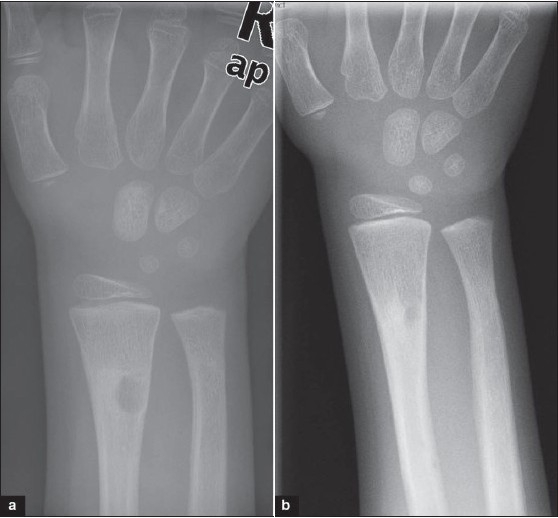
Anteroposterior X-ray (a) of forearm with wrist at 3 month follow-up shows a well defined cystic lesion at the original site of the lesion within the newly formed subperiosteal bone. (b) X-ray at six month follow-up shows complete resolution of the cyst.
